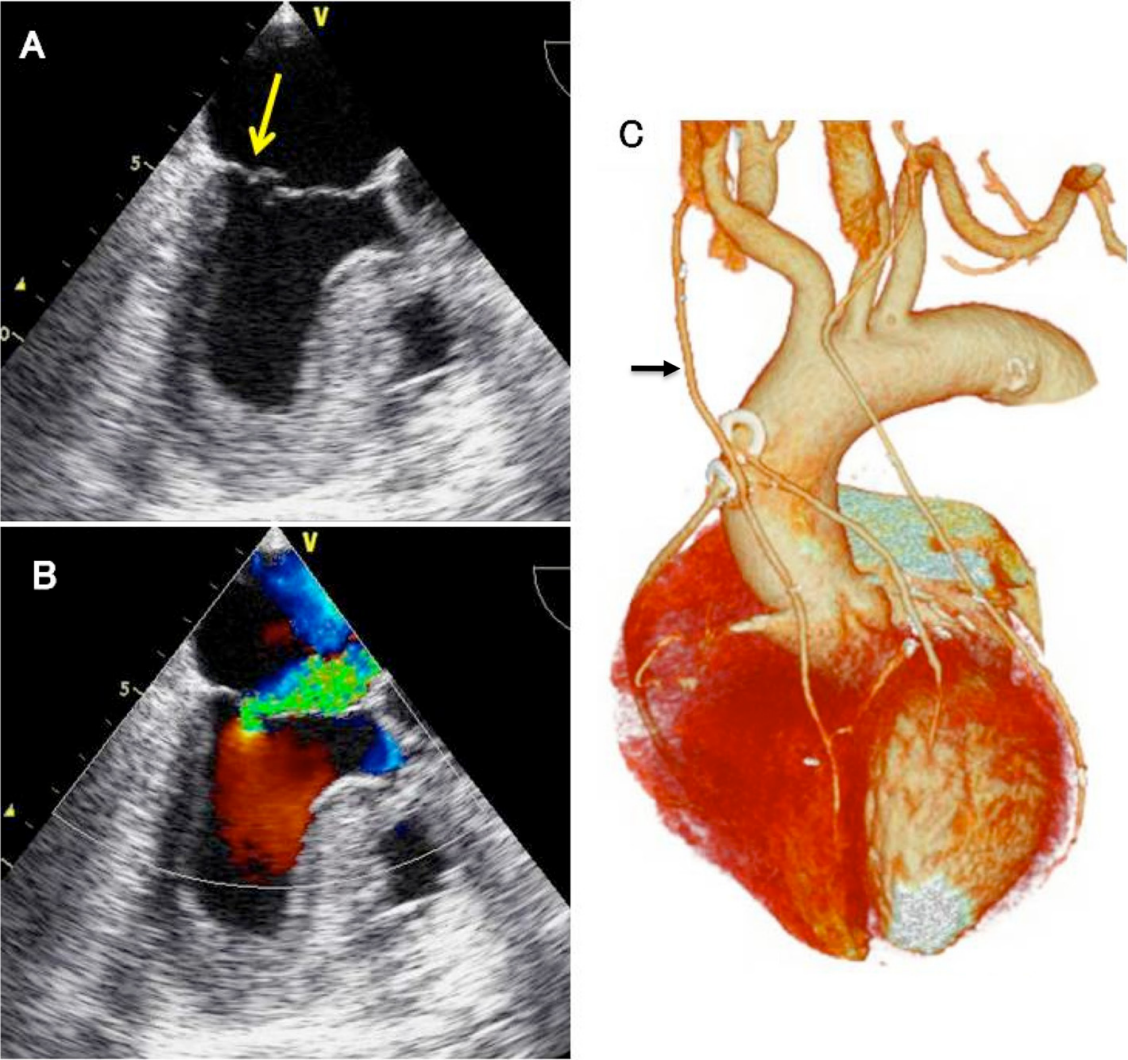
Preoperative images of the presented case. (A) Echocardiographic image showing prolapse of the posterior leaflet of the mitral valve (arrow). (B) Color Doppler image showing severe mitral regurgitation. (C) Computed tomographic scan showing the patent bypass grafts. Note that the right internal mammary artery (arrow) runs across the ascending aorta.
